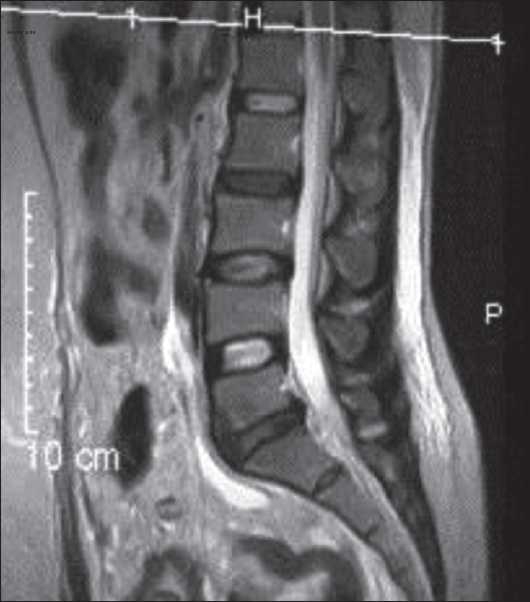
Normal magnetic resonance imaging
